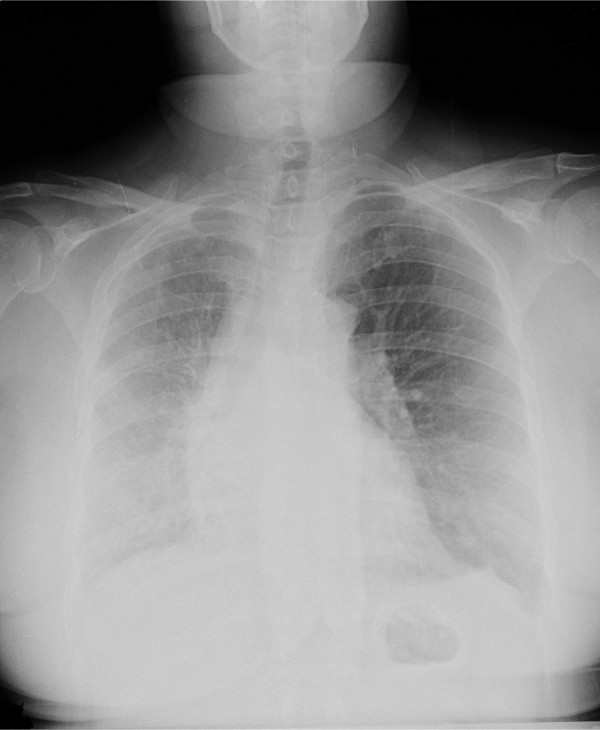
Chest radiograph showing loss of volume of her right lung with displacement of the mediastinum to the right.
